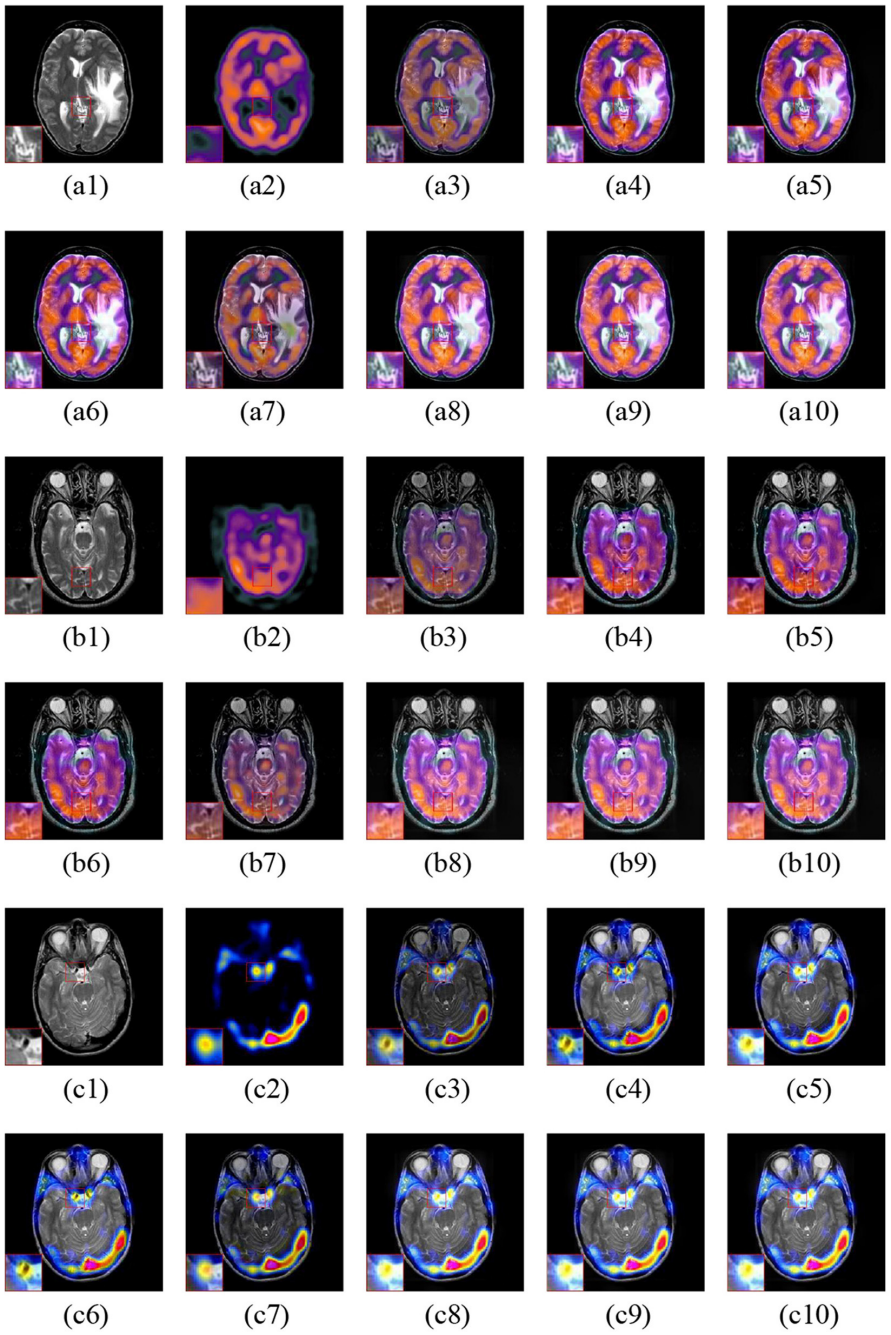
Three fusion examples of MR and SPECT images. One close-up is provided in each set for better comparison. The original images: (a1–c1) MR and (a2–c2) SPECT. The fusion results: (a3–c3) ASR, (a4–c4) NSCT-PC-LLE, (a5–c5) LP-CNN, (a6–c6) NSST-PAPCNN, (a7–c7) CSMCA, (a8–c8) LatLRR-FCN-32s, (a9–c9) LatLRR-FCN-16s, and (a10–c10) LatLRR-FCN-8s.
